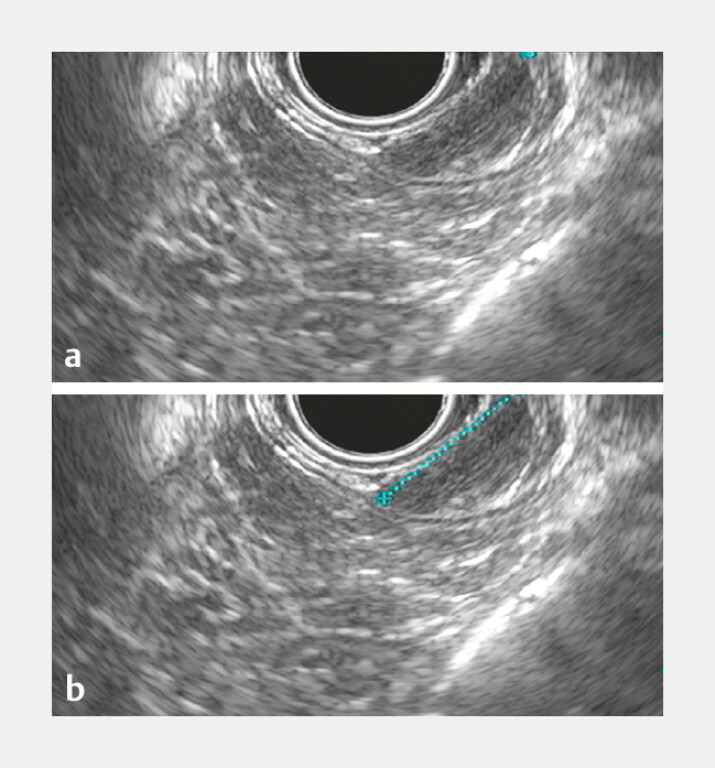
Endoscopic ultrasound (EUS) exposed an image with a hypoechoic echotexture, homogeneous, measuring approximately 13 mm, with precise limits, regular contours, and inserted in the submucosal layer.
